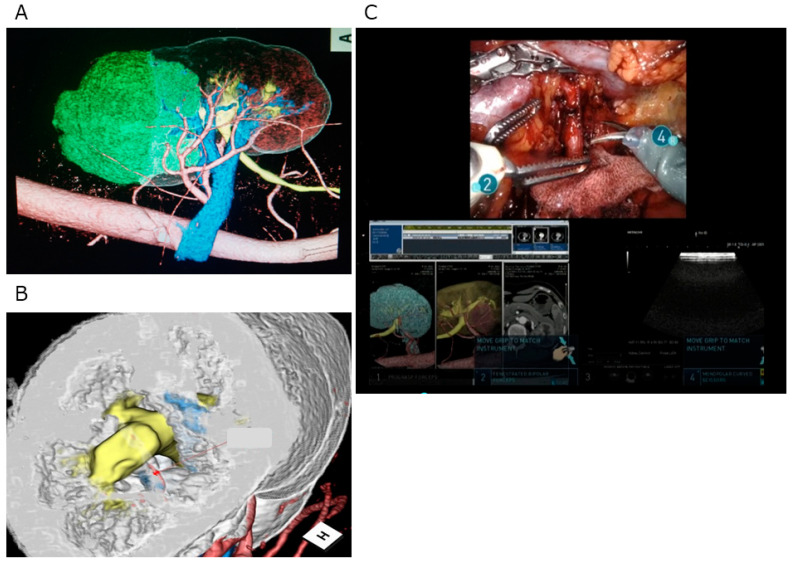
Intraoperative navigation system using ultra-high-resolution computed tomography. (A) Image of the tumor, urinary tract, and vascular structures, including the renal pedicle. (B) Preoperative image of the cut surface of the tumor. (C) Intraoperative navigation system using TilePro software. (A) and (B) show the same renal tumor; (C) shows another renal tumor.
